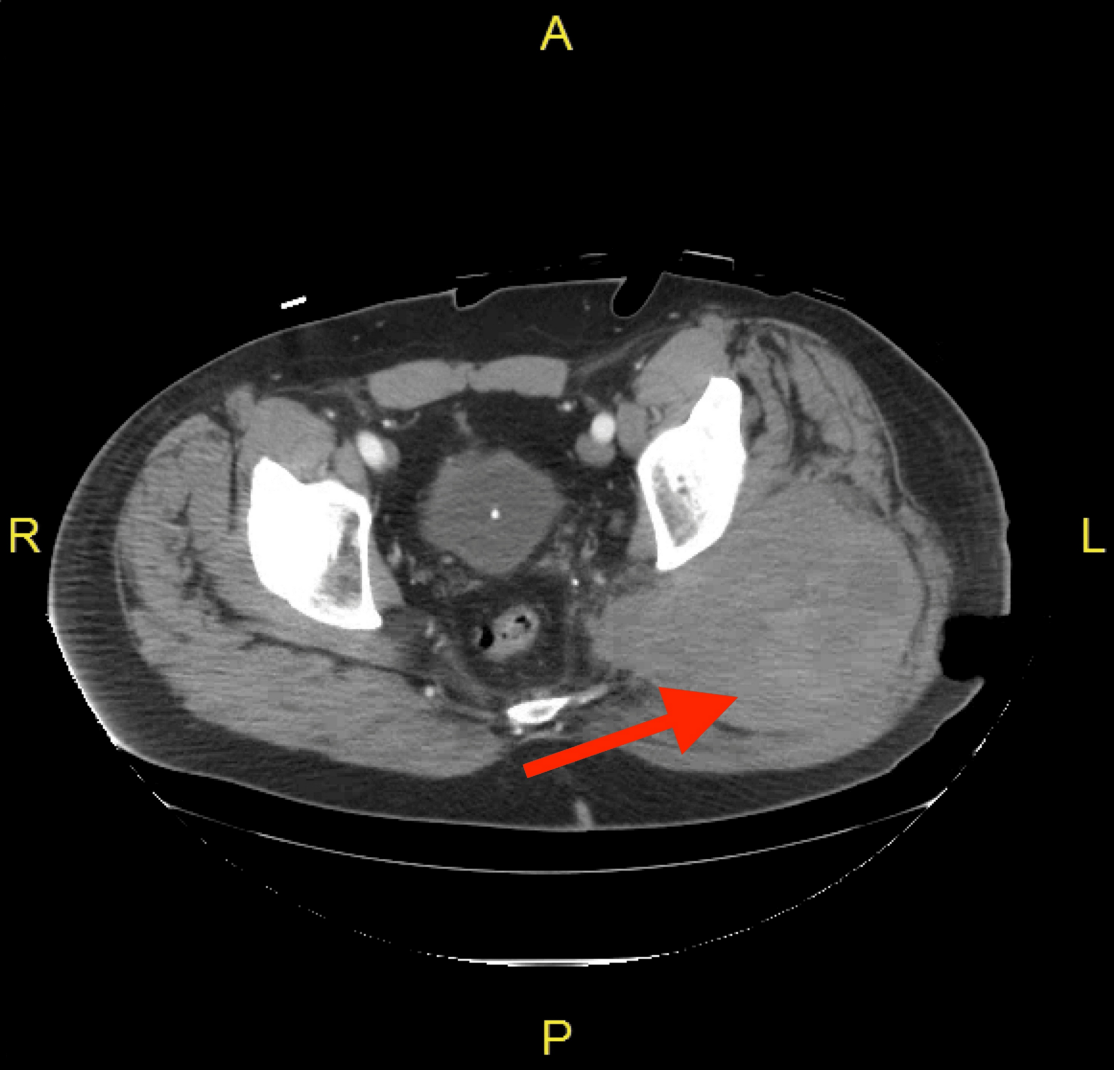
Abdominal/pelvis CT angiography (axial view) No acute fractures. A large, well-circumscribed hyperdense mass (red arrow), measuring 12.7 x 14.3 cm, is seen posteriorly to the left hip. The impression is of a large hematoma posterior to the left hip joint, with active enhancement consistent with active hemorrhage.
